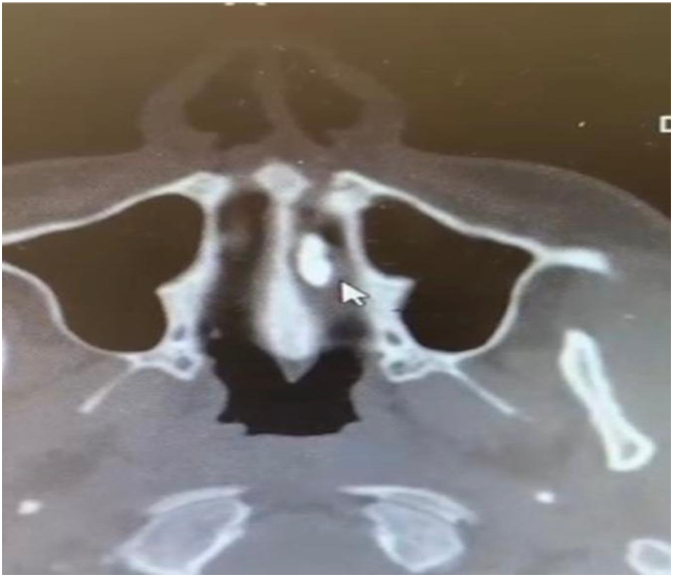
CT scan axial cut bone window showing the nasal tooth.
